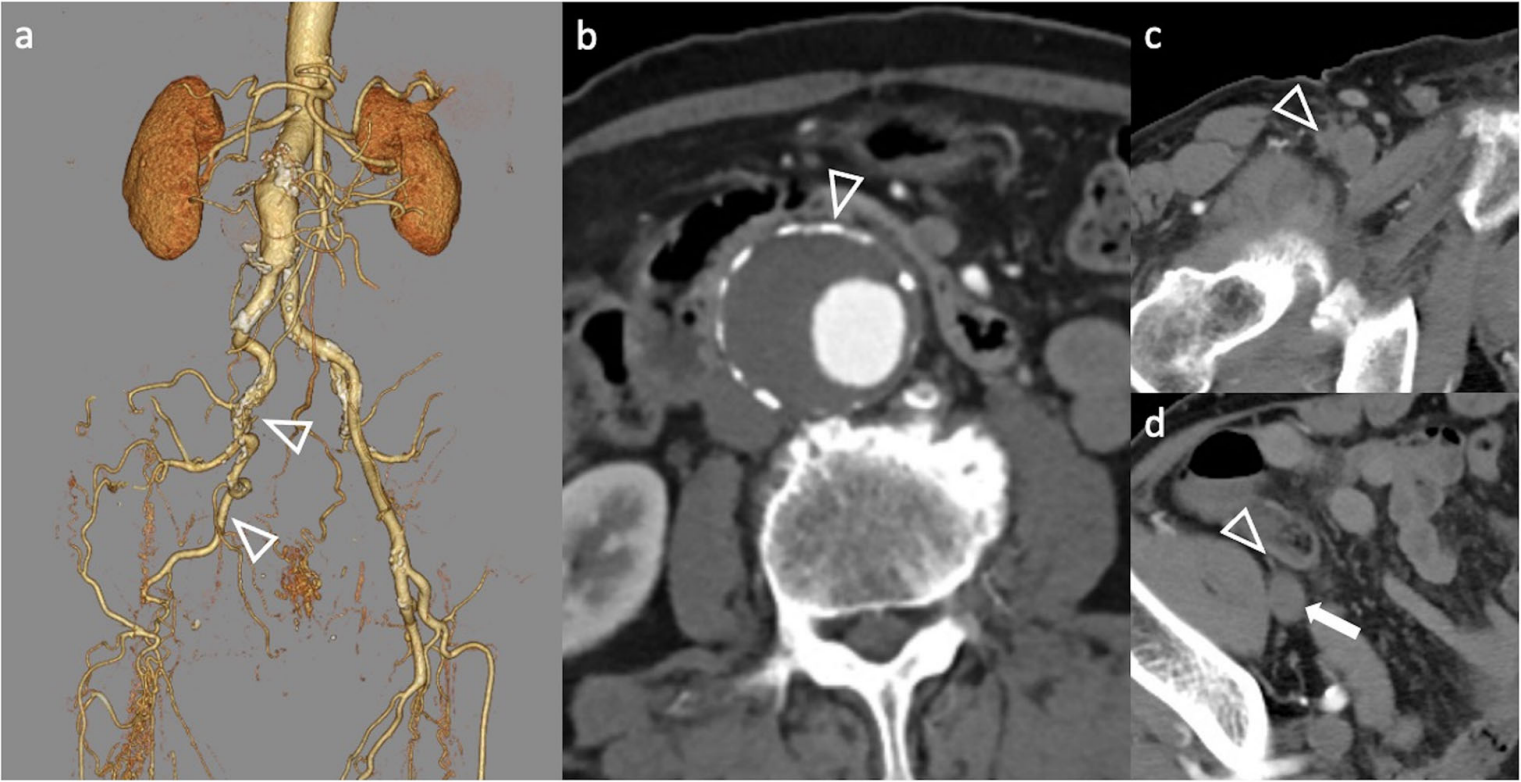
CT before endovascular aneurysm repair. a VR image of CT angiography shows the occlusion of the right EIA to the SFA with collateral network from the right hypogastric artery (arrow heads), b Axial plane of CT shows AAA (arrow head), c Contrast enhanced CT shows occlusion of the right CFA (arrow head), d The occluded EIA (arrow head) was atrophied. Arrow shows EIV. CFA: common femoral artery, EIA: external iliac artery, EIV: external iliac vein, SFA: superficial femoral artery, VR: volume rendering
